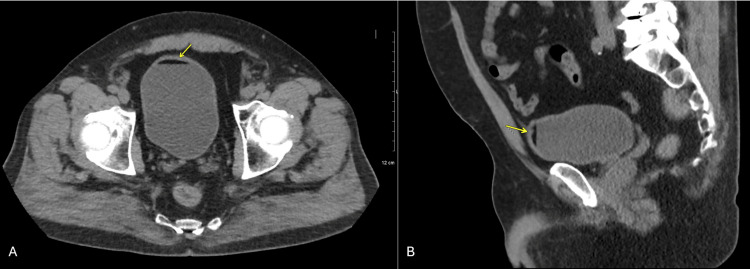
Follow-up CT with chyluria, axial (image A) and sagittal (image B) Fat-fluid level highlighted with yellow arrows on both axial (image A) and sagittal (image B), pathognomic for chyluria
